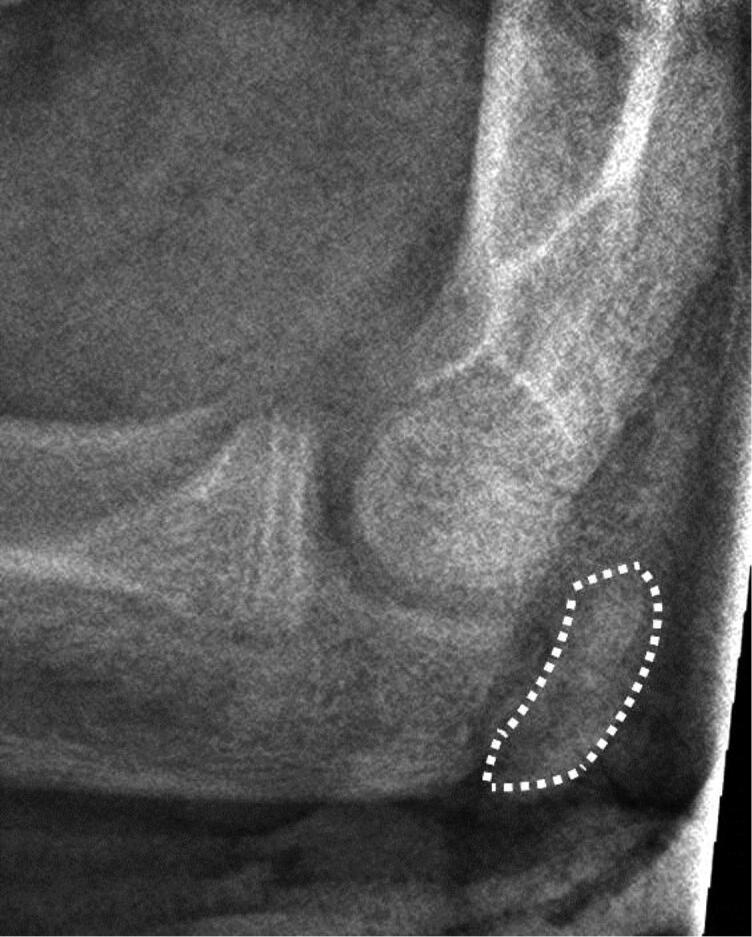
The elbow has been captured on the lateral view of the forearm radiograph and the secondary ossification center of the olecranon has been marked with a border (dotted line). The patient is a male aged 12. According to the Sauvegrain and Dimйglio method the olecranon ossification stage with a rectangular shape is 6. This stage was found to be a cutoff point in association with disturbed bone union after ESIN of forearm shaft fracture.
